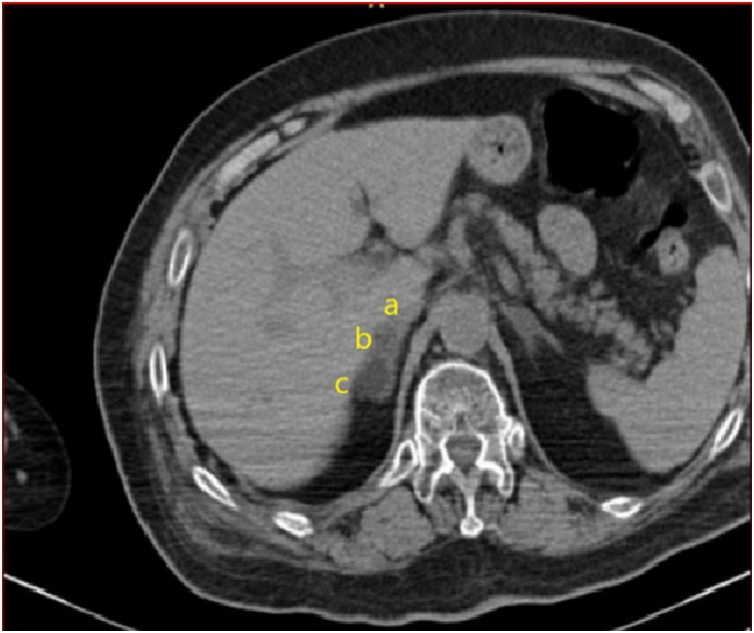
Plain abdominal CT follow-up imaging after three days shows a distinct hypodense area of the non-traumatic oval lesion and a smaller posterior hyperdense area (55 HU) of the gland close to the liver. Notice the three different density areas around the gland (a,b,c).
